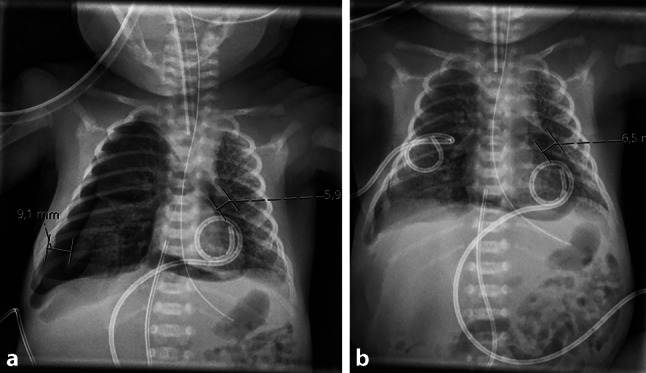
a Chest X-ray demonstrating right-sided pneumothorax, b Chest X-ray after insertion of a chest tube (pigtail) catheter
